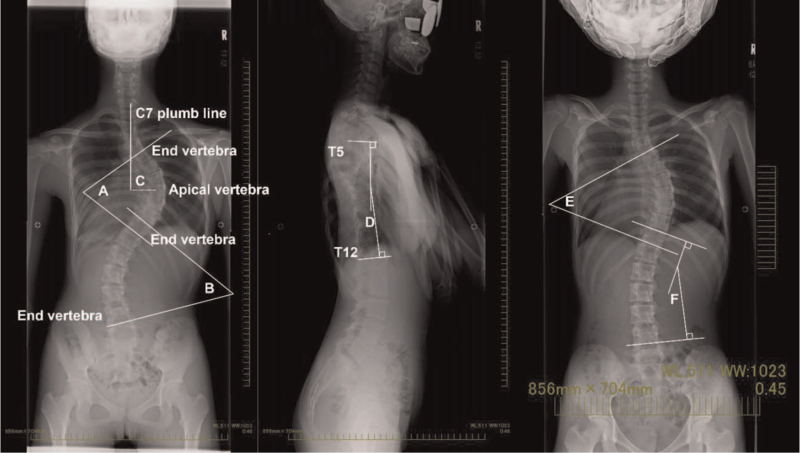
X-ray measurements of coronal and sagittal parameters. The Cobb angles, A and B, are angles between the upper and lower end vertebra of each thoracic and lumber curvature. The apical vertebra translation of thoracic curvature, C, is the distance between the midpoint of the apical vertebra and C7 plumb line. The thoracic kyphosis, D, is the angle between upper endplate of T5 and lower endplate of T12. The Cobb angles with manual traction in supine position, E and F, are the angles between upper and lower endplates of end vertebra of each thoracic and lumber curvature.
